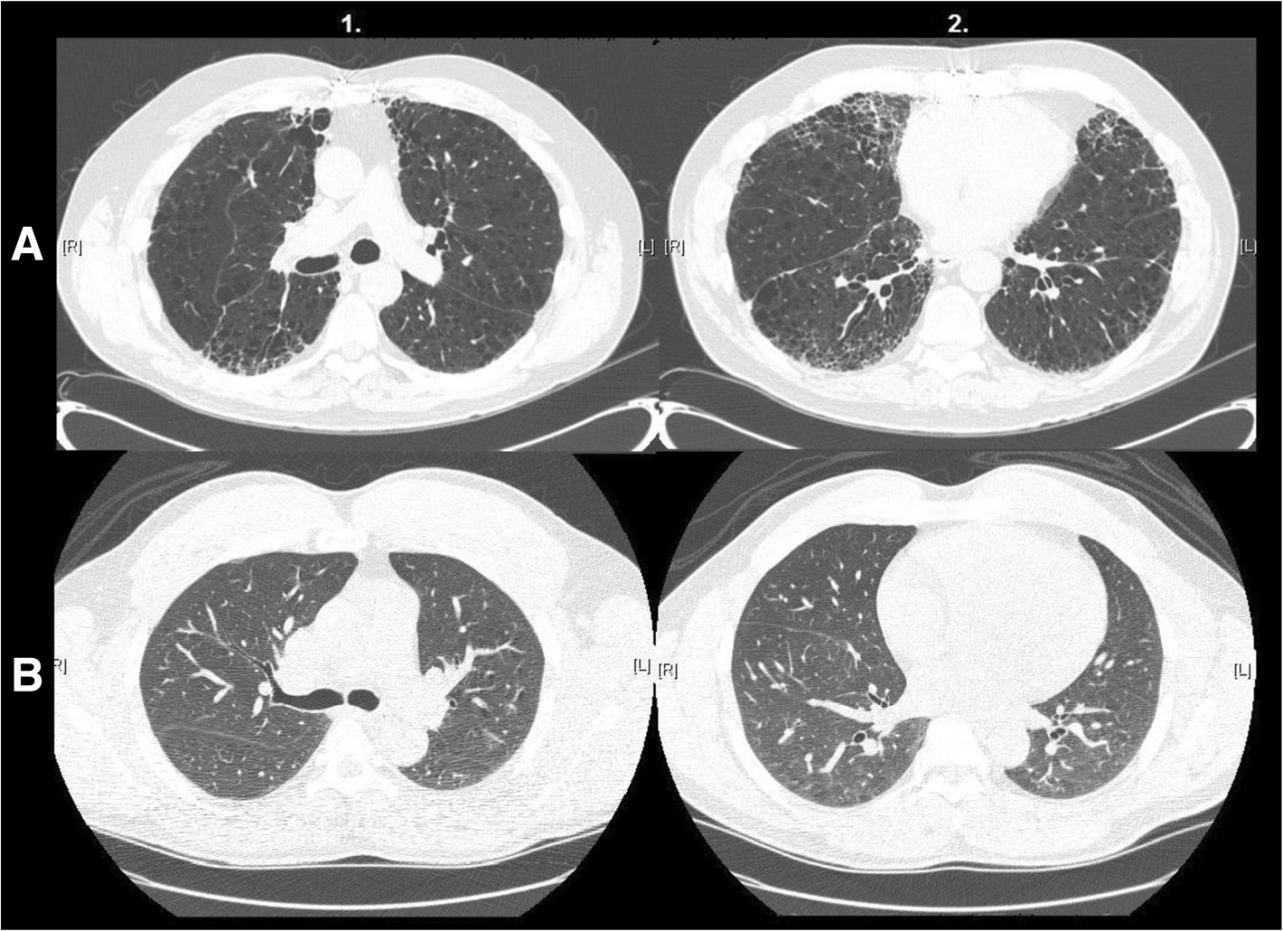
On the vertical axis we present representative examples of (a) a subject with interstitial lung abnormalities identified by visual assessment but has less than 10 % of the lung with chest CT attenuation values between −600 and −250 HU (HAA) (7.5 % HAA) and (b) a subject having > 10 % of the lung with chest CT attenuation values between −600 and −250 HU (HAA) (10.8 %) but not identified as having interstitial lung abnormalities identified by visual assessment. Each row represents data from a single subject. On the horizontal axis we present axial high resolution chest computed tomographic (HRCT) images (1 [approximately at the level of the carina] and 2 [approximately at the level of the right inferior pulmonary vein])
